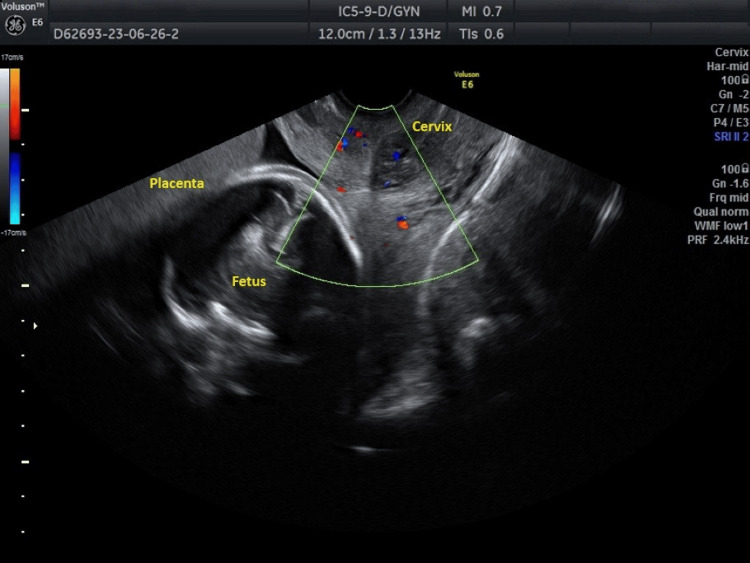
Transvaginal Doppler ultrasound imaging suggesting vasa previa during the second trimester of pregnancy The presence of blood flow near and around the internal cervical os of the uterus raises strong suspicion for the presence of vasa previa.
